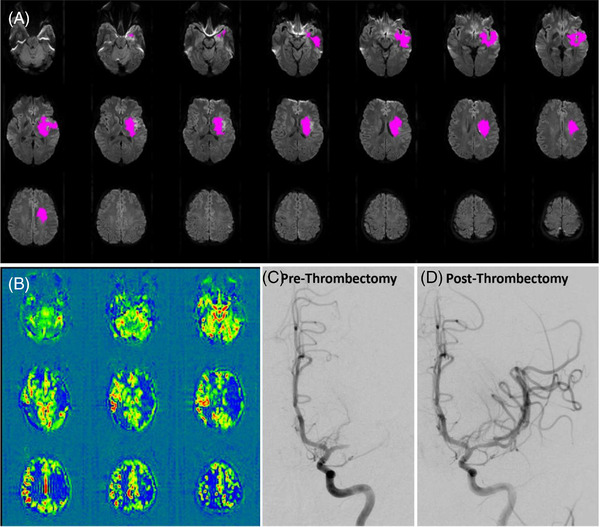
Relevant neuro‐imaging showcasing key points in the case. A, MRI brain with perfusion imaging, performed after aggressive resuscitation in the ICU, demonstrated an approximately 45cc core infarct (pink). B, The corresponding ASL perfusion map demonstrated a perfusion deficit in the left MCA territory that was significantly larger than the identified core. C, Cerebral angiogram prethrombectomy, (D) cerebral angiogram postthrombectomy of a left M1 thrombus with TICI‐2b reperfusion. ASL indicates arterial spin labeling; ICU, intensive care unit; MCA, middle cerebral artery; MRI, magnetic resonance imaging; and TICI, thrombolysis in cerebral infarction.
